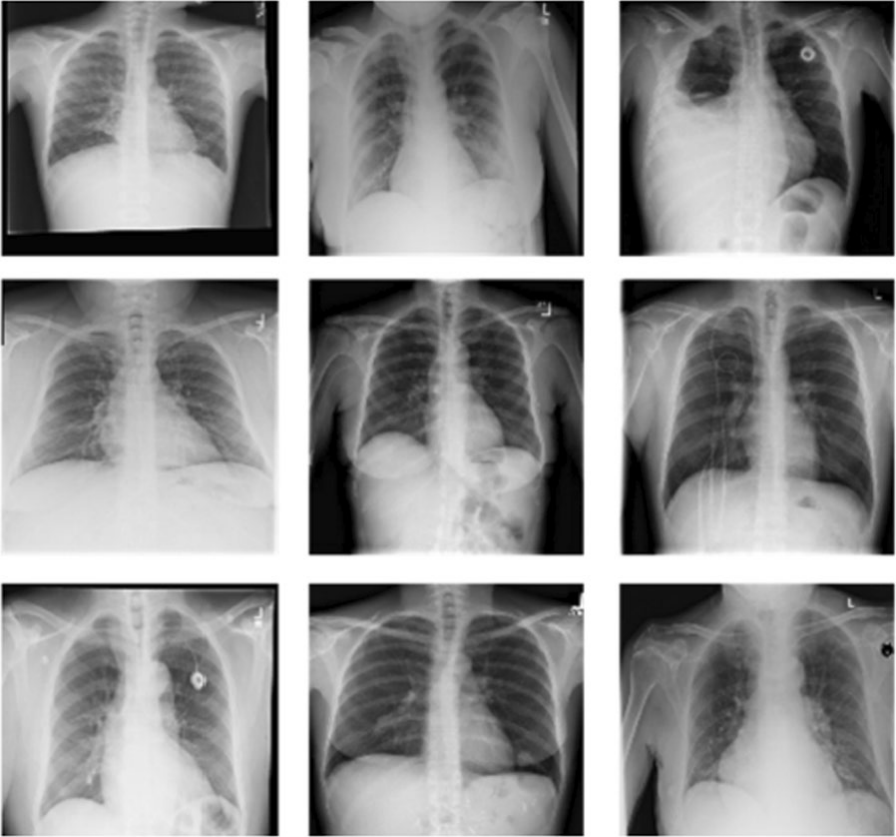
Samples from the NIH chest X-ray dataset
